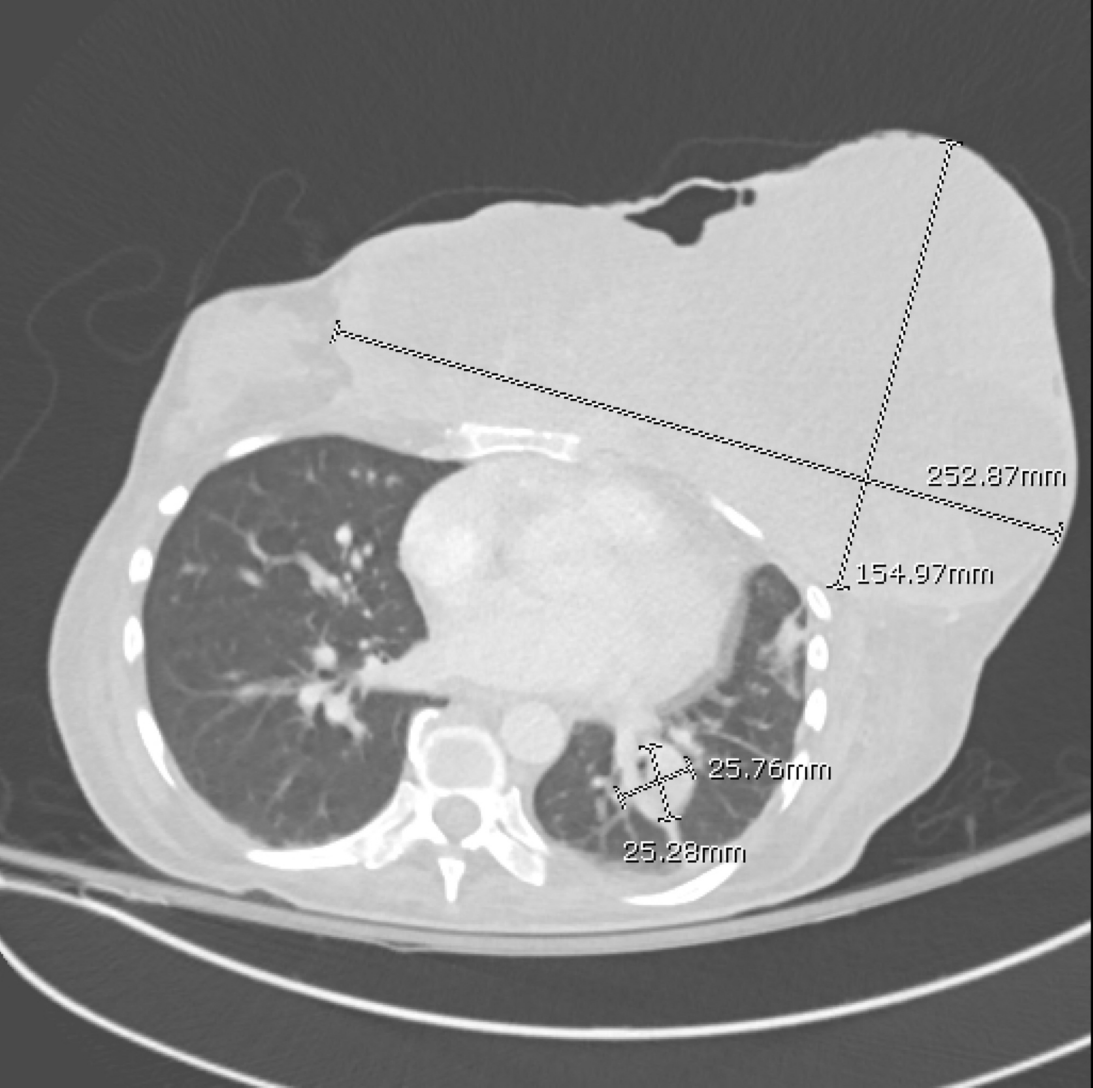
CT chest axial view showing extensive primary tumor burden as well as lung metastasis CT: computed tomography
